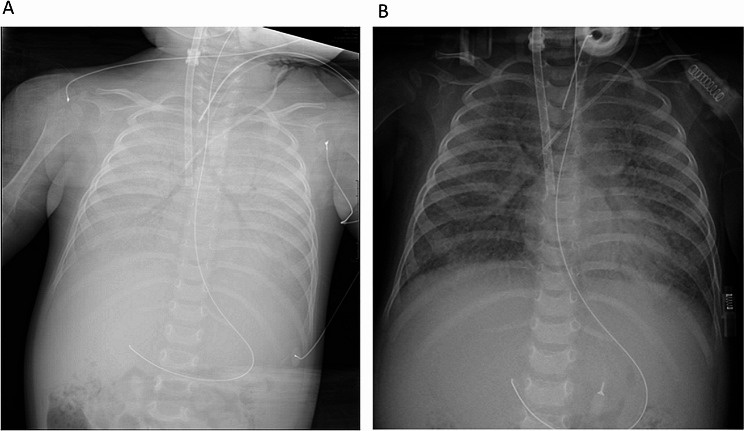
Chest X-ray prior (A) and after (B) the first whole lung lavage. Note the diffuse reticulogranular opacity (A)
